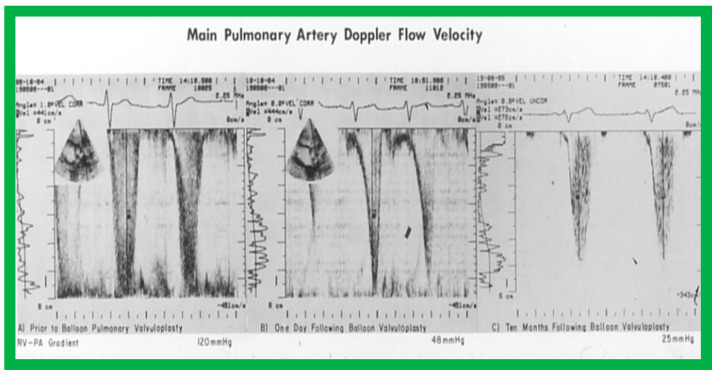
The main pulmonary artery Doppler flow velocities prior to (A), one day (B), and ten months (C) following balloon pulmonary valvuloplasty are shown. Note that there is a significant fall in the peak flow velocity immediately after valvuloplasty, but a moderate (48 mmHg) gradient that has a characteristic triangular pattern, highly suggestive of infundibular obstruction (corresponding to Figure 20 left) persisted. At the 10-month follow-up, the flow velocity has markedly diminished, indicating the resolution of the infundibular obstruction (corresponding to Figure 20 right). The residual calculated gradients are shown at the bottom of each panel. Reproduced from Reference [69].
